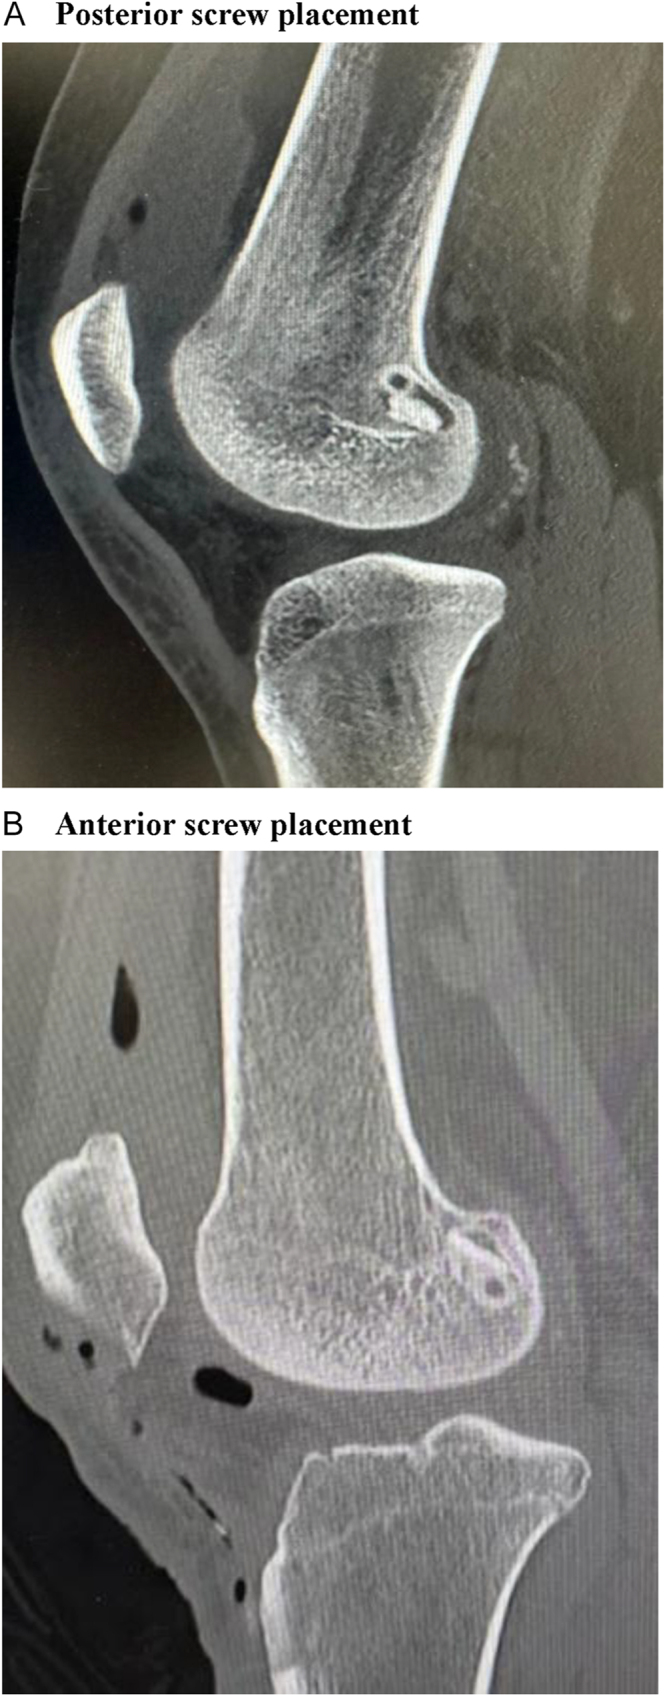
Sagittal view of CT showing BTB fixed with an absorbable interference screw: (A) posterior screw placement and (B) anterior screw placement.
